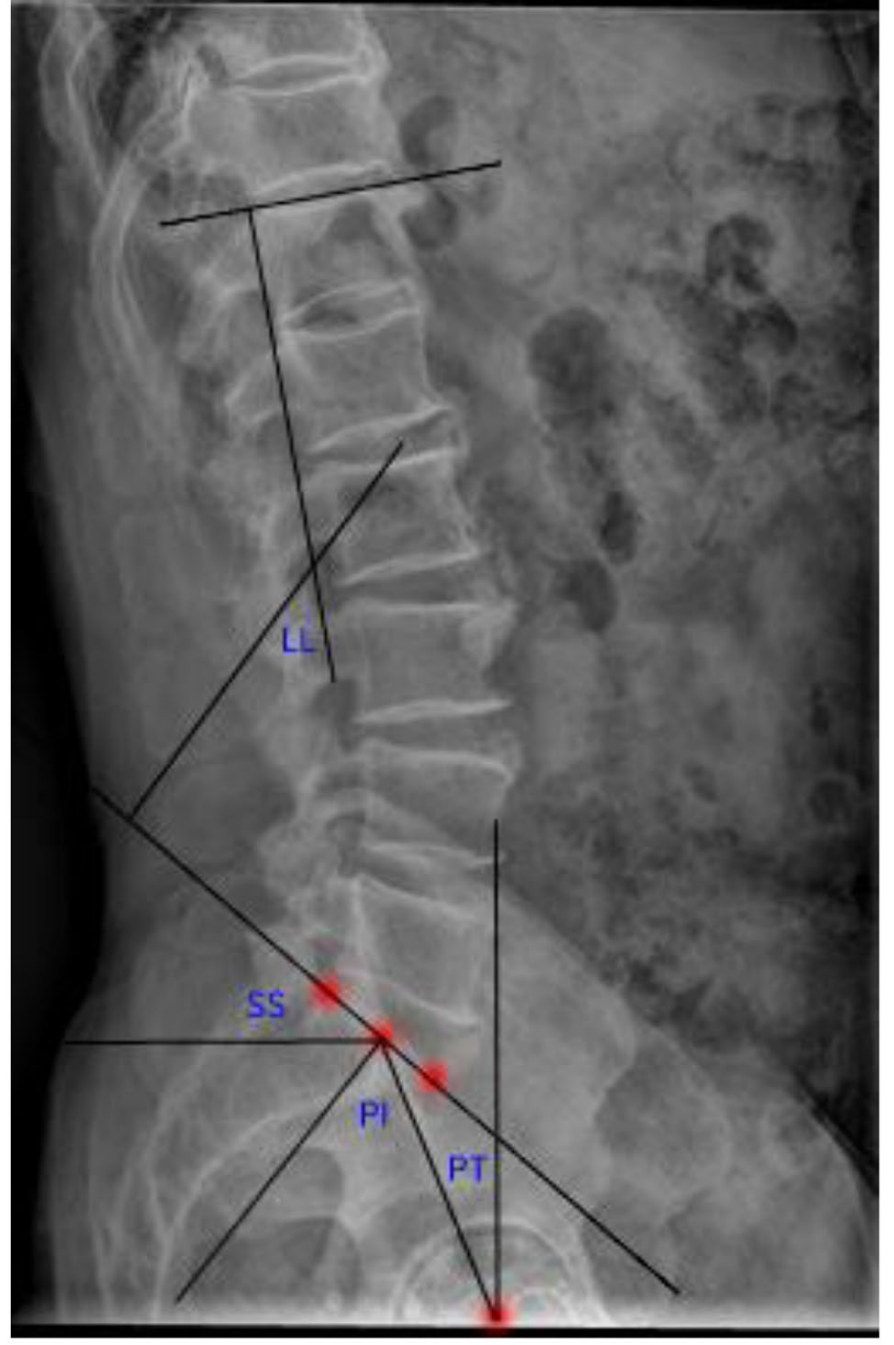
Plain lateral radiographs for measuring spinal-pelvic sagittal plane parameters. PI: Pelvic incidence; PT: Pelvic tilt; SS: Sacral slope; LL: Lumbar lordosis
